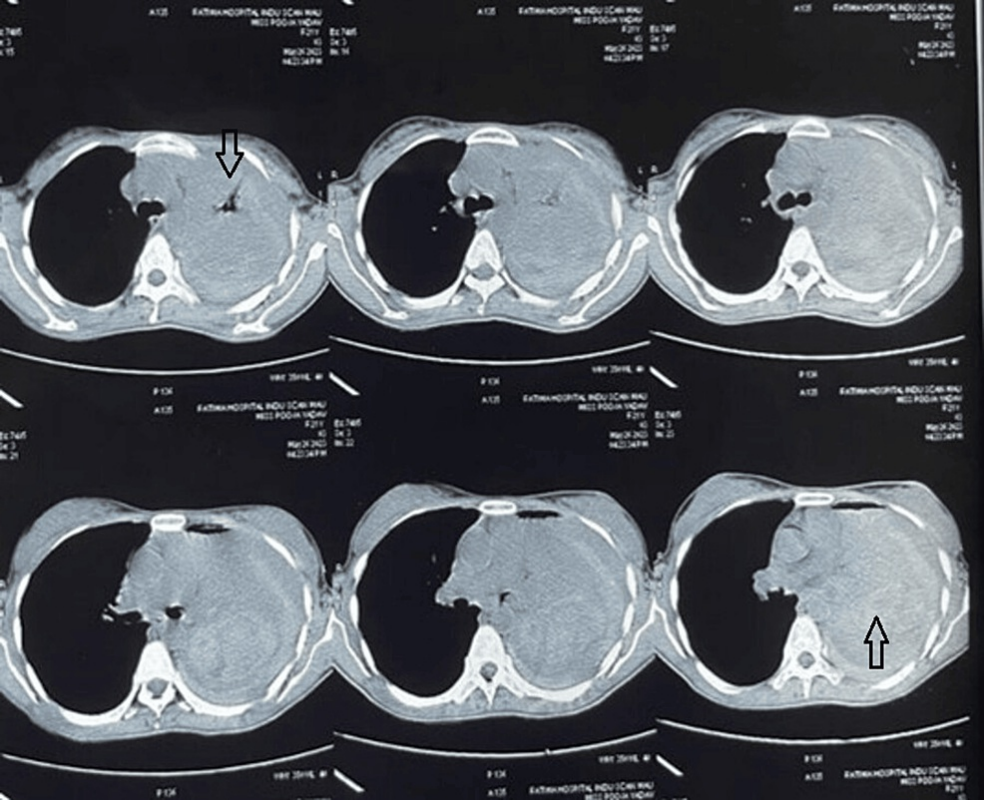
High-resolution computed tomography (HRCT) thorax showing left-sided pneumothorax with multiple membranes, thick septations, gross left-sided pleural effusion and collapse of the underlying lung parenchyma (arrow)
